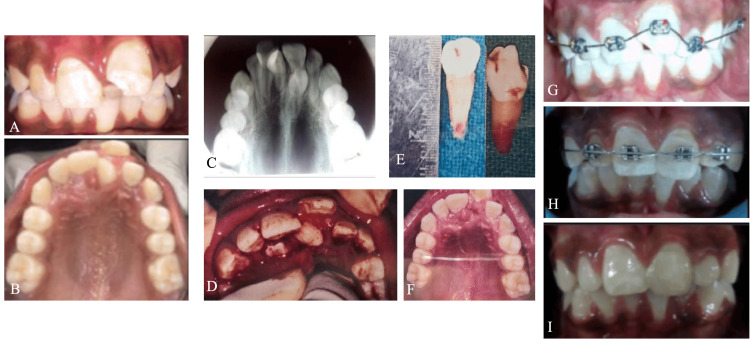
Case 2 A: pre-operative frontal view; B: pre-operative maxillary occlusal view; C: pre-operative maxillary occlusal intraoral periapical radiograph (IOPA); D: flap raised and extraction of mesiodentes; E: extracted mesiodentes; F: post-operative maxillary occlusal view; G,H: two-by-four appliance therapy; I: post-operative frontal view
